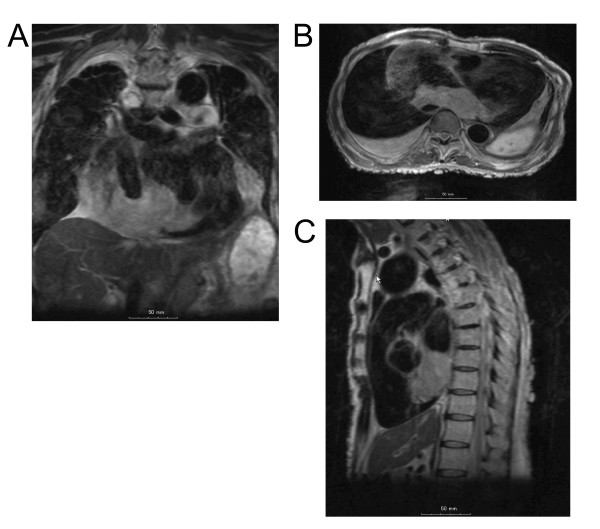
Magnetic resonance imaging showed a large tumor in the inferior-posterior wall of the heart. (A) Frontal cross section. Size: 7.5 cm. (B) Horizontal cross section. Size: 9 cm. (C) Sagittal cross section. Size: 6.5 cm.
